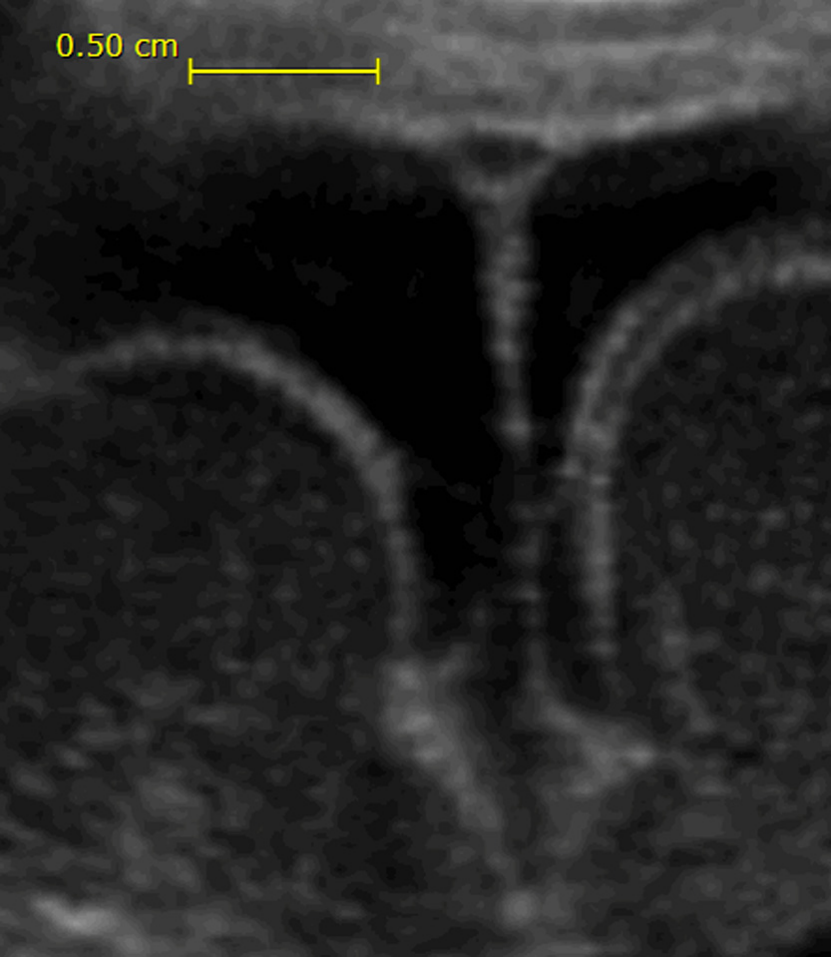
Cross-sectional image of the SSS in a 27-week gestation preterm infant at the level of the anterior fontanel. Although ultrasound can penetrate bone, good images require the fontanel as an acoustic window. The black layer between the inner surface of the skull/fontanel and the surface of the brain is unusually wide in this infant, about 7 mm, and is constituted by cerebrospinal fluid in the subarachnoidal space. The subarachnoidal space separates the two brain hemispheres in a deep V-shape. The thin line in the middle represents the falc cerebri. On top of this, the triangular SSS is seen, measuring 2 to 3 mm on each side. It is black on the ultrasound image, since flowing blood, such as cerebrospinal fluid, gives few echoes to ultrasound.
